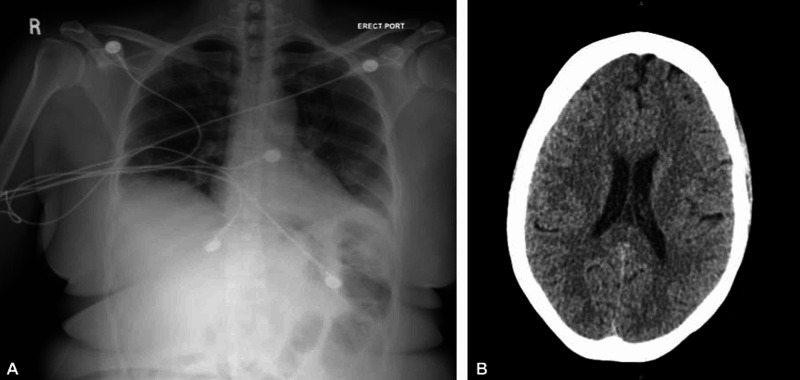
A. Anteroposterior view chest X-ray at the patient’s second ED visit; B. CT of the head without contrast at the patient’s second ED visit A. This image does not reveal any typical active COVID-19 radiologic signs. There was a limited inspiratory effort, no pulmonary vascular congestion or edema, no pulmonic infiltration, no atelectasis or pleural effusions demonstrated. The cardiomediastinal silhouette is normal in size and configuration, with the bony thorax intact. B. There are no signs of acute intracranial hemorrhage, acute infarct in a major vascular territory, or mass-effect. ED: emergency department; CT: computed tomography
